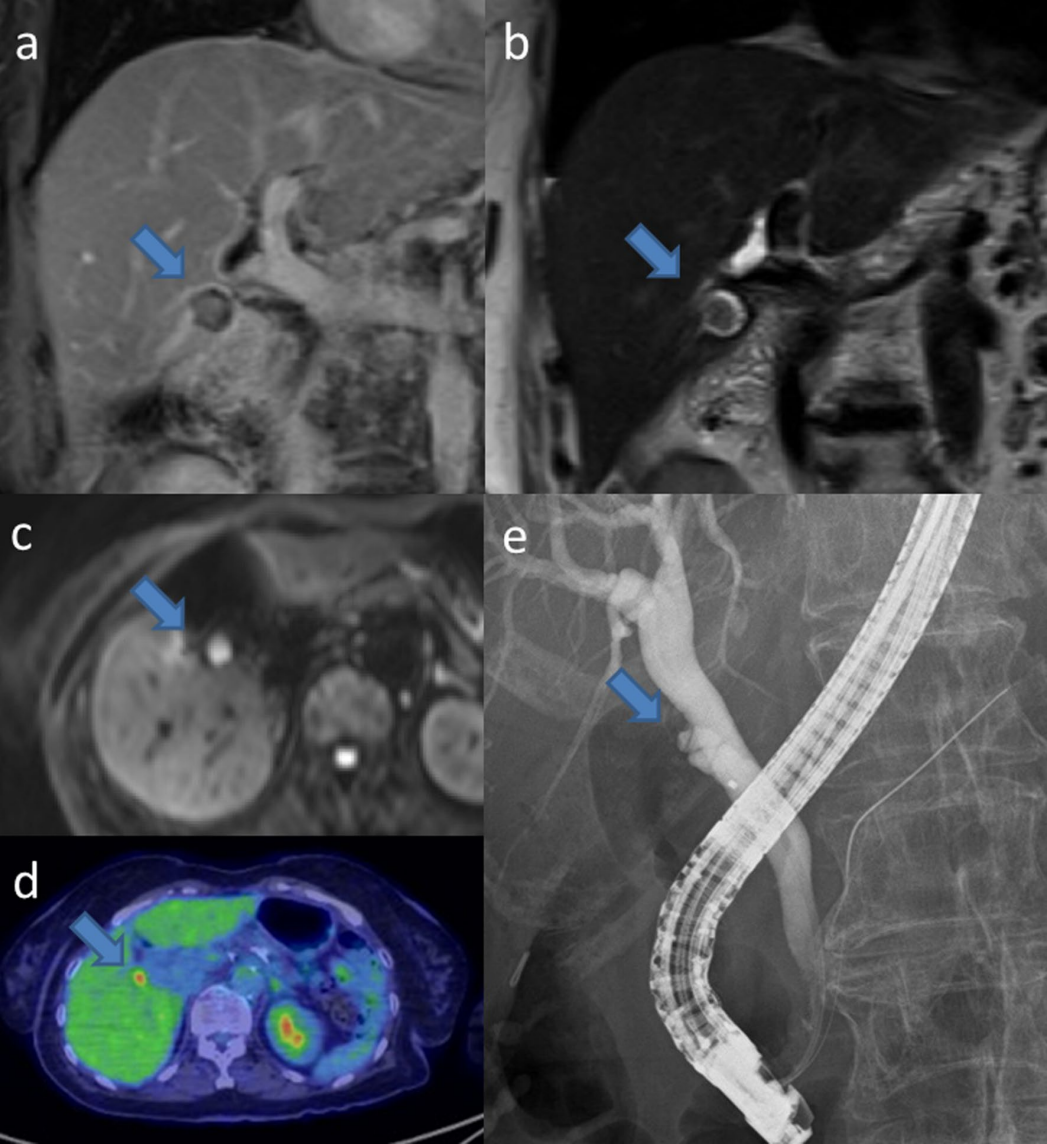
Findings of additional imaging studies. a T1-weighted magnetic resonance imaging (MRI). A 10-mm enhanced nodule is visible (arrow) without evidence of extramural invasion. b T2-weighted MRI. The lesion is detected as a filling defect (arrow). c Diffusion-weighted MRI. Restricted diffusion of the lesion is observed (arrow). d 18-fluoro-2-deoxy-glucose positron emission tomography/computed tomography (FDG-PET/CT). FDG accumulation (maximum standardized uptake value: 6.90) in the remnant gallbladder lesion is observed (arrow). e Endoscopic retrograde cholangiopancreatography. The cystic duct, which diverged from the left side of the middle bile duct, was confirmed. Findings suspicious for invasion to the bile duct and pancreatobiliary maljunction are not observed. Contrast medium did not flow into the remnant gallbladder via the cystic duct (arrow). The length of the intact cystic duct was approximately 15 mm. According to the cholangiography findings, extrahepatic bile duct resection was planned to be omitted
